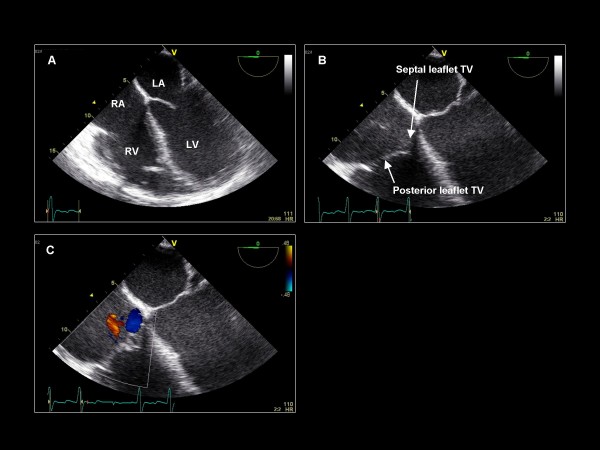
Imaging plane 0°, (A) The mid-esphageal four chamber view. (B) The tricuspid valve anatomy with septal and posterior leaflets. (C) Color Doppler applied to the tricuspid valve demonstrating trivial tricuspid regurgitation (orange jet). LA = left atrium, LV = left ventricle, RA = right atrium, RV = right ventricle, TV = tricuspid valve.
